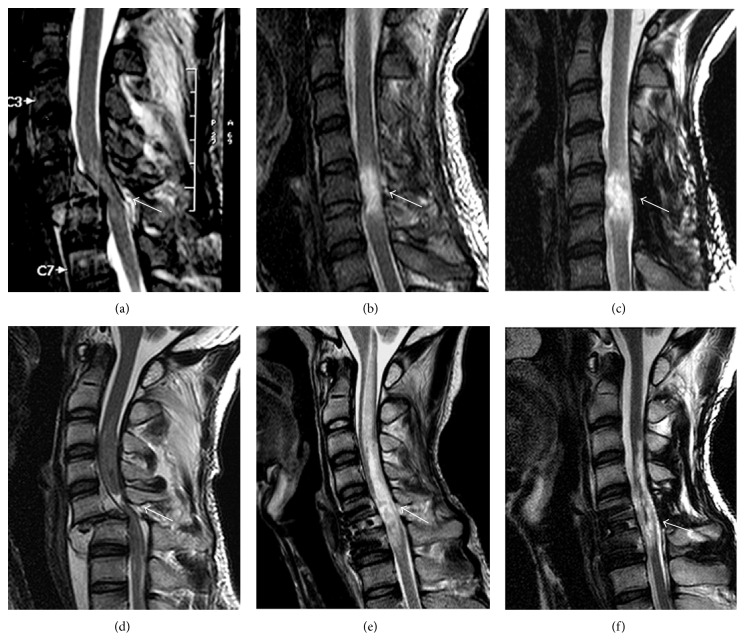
Sagittal T2W MRI scan of two SCI patients (upper panel: patient 8; lower panel: patient 15) at the time of injury (a, d), before transplantation as a baseline (b, e), and 1 year after transplantation (c, f). Follow-up findings showed progressive myelomalacic change at the site of cell transplantation (c) or myelomalacia and atrophy of the cord (f) in cell implantation areas. The white arrows mark the site of injury.
